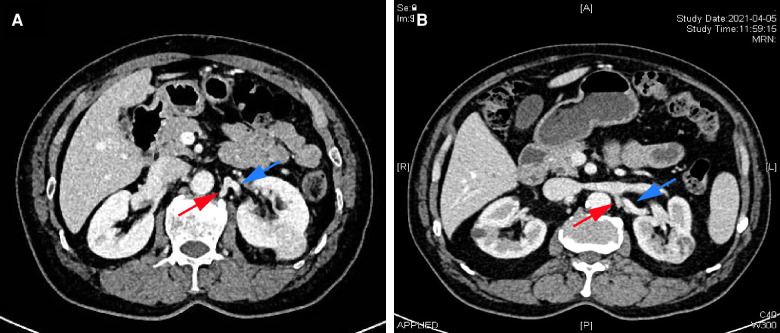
Perioperative computed tomography (CT) scans from two patients with renal cancer patients. (A,B) Renal contrast-enhanced CT demonstrating the lumbar vein (red arrow) and the left renal artery (blue arrow).
